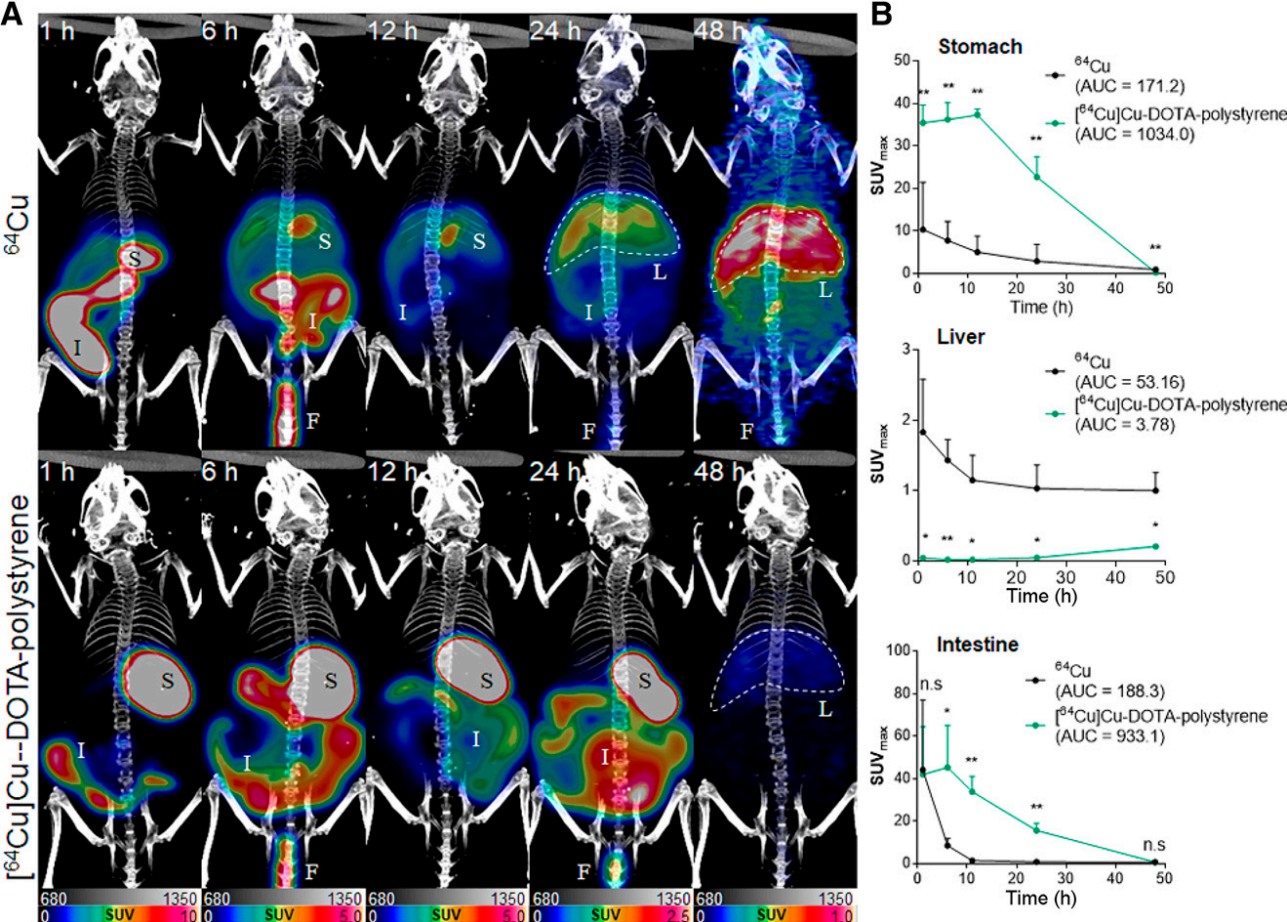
(A) Representative PET/CT of orally administered [^64^Cu]Cu-DOTA-polystyrene or ^64^Cu. [^64^Cu]Cu-DOTA-polystyrene accumulated in stomach and intestine for 24 h. Uptake of [^64^Cu]Cu-DOTA-polystyrene was observed in liver at 48 h after administration. However, ^64^Cu in stomach and intestinal track was rapidly cleared and transported to liver. (B) SUV_max_ of [^64^Cu]Cu-DOTA-polystyrene was significantly higher than that of ^64^Cu in stomach and intestine. In contrast, SUV_max_ of [^64^Cu]Cu-DOTA-polystyrene was significantly lower than that of ^64^Cu in liver. F = feces; I = intestine; L = liver; S = stomach. *n* = 5. P* < 0.05, Student *t* test. *P* < 0.005, Student *t* test.
